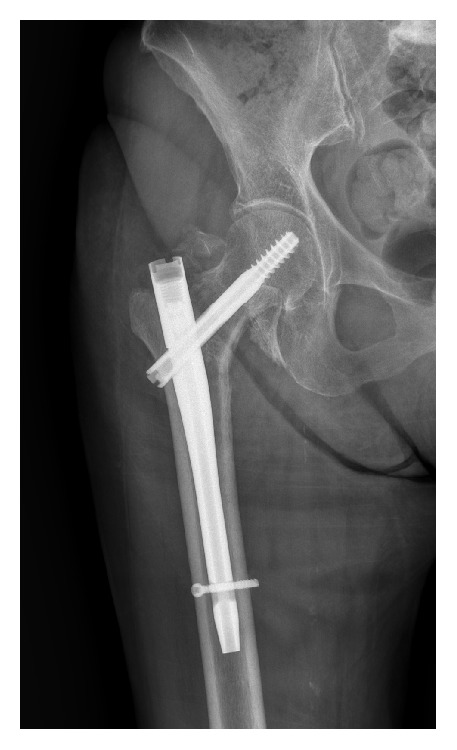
The AP view radiograph of the right hip demonstrates proximal migration of the lesser trochanter.
